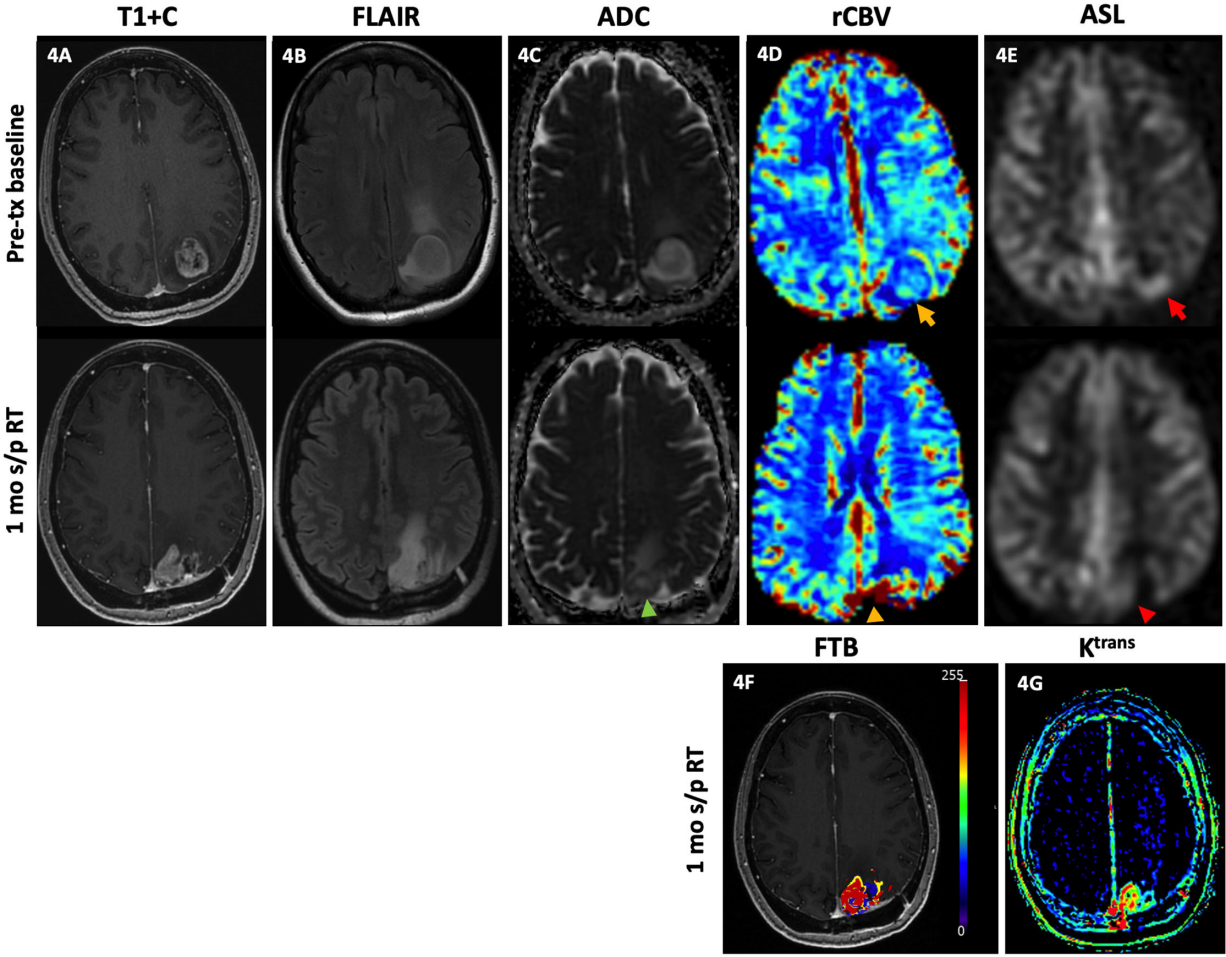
Tumor recurrence. A 49-year-old female with GBM (IDH wt, MGMT+) was initially diagnosed with an enhancing left parietal mass [(A), top row] with associated lesional and perilesional FLAIR hyperintensity [(B), top row], diffusion restriction [(C), top row], increased rCBV [(D), top row, yellow arrow], and ASL hyperperfusion [(E), top row, red arrow]. She then underwent gross total resection and concurrent CRT with minimal residual enhancement of the resection cavity margins without nodular enhancement, diffusion restriction, or increased rCBV or ASL signal (not shown). One month after completion of RT, there is new focal nodular enhancement at the medial aspect of the resection bed [(A), bottom row] with associated FLAIR hyperintensity [(B), bottom row], diffusion restriction [(C), bottom row, green arrowhead], increased rCBV [(D), bottom row, yellow arrowhead], and ASL hyperperfusion [(E), bottom row, red arrowhead]. There is increased FTB_high_, within the lesion as reflected by the red-colored areas (F), and increased vascular permeability on the K^trans^ map (G). Note on the rCBV, FTB, and K^trans^ maps, a color scale of blue to red represents the incremental increase in values from low to high. The findings of increased enhancement, FLAIR hyperintensity, diffusion restriction, rCBV, FTB_high_ and K^trans^ favor recurrence. Subsequent resection of the lesion showed recurrent GBM. ADC, apparent diffusion coefficient; ASL, arterial spin labeling; FLAIR, fluid-attenuated inversion recovery; FTB, fractional tumor burden; GBM, glioblastoma; IDH, isocitrate dehydrogenase; K^trans^, volume transfer coefficient; MGMT, O-6-methylguanine-DNA methyltransferase; mo, months; rCBV, relative cerebral blood volume; RT, radiation therapy; s/p, status post; T1+C, post-contrast T1-weighted images; tx, treatment; wt, wildtype.
